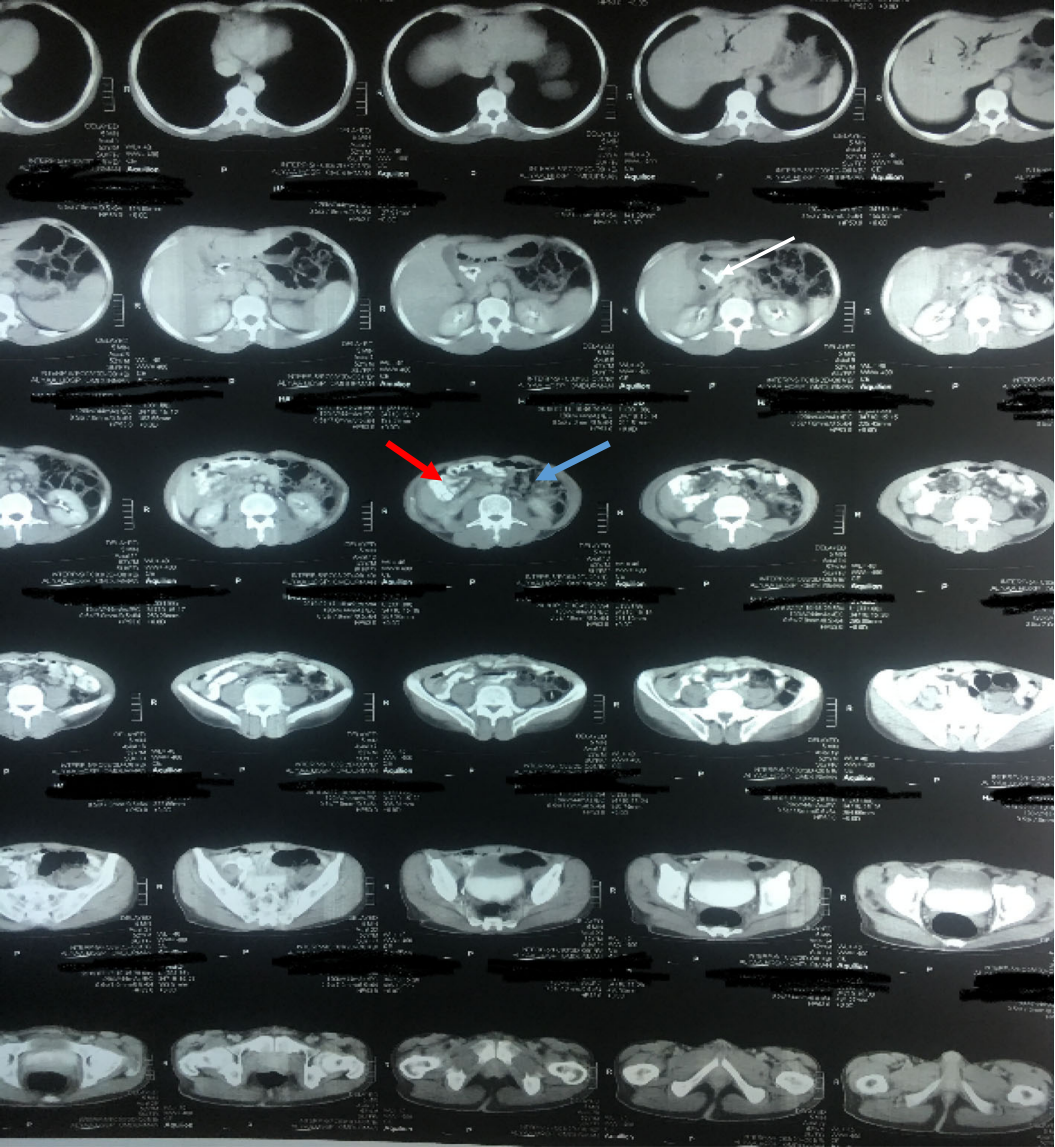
Computed tomographic scan of the abdomen showing malrotation of the bowel, periampullary mass, dilated common bile duct, and stent inside (white arrow), small bowel on the Right side (red arrow) and large bowel on the left (blue arrow)
